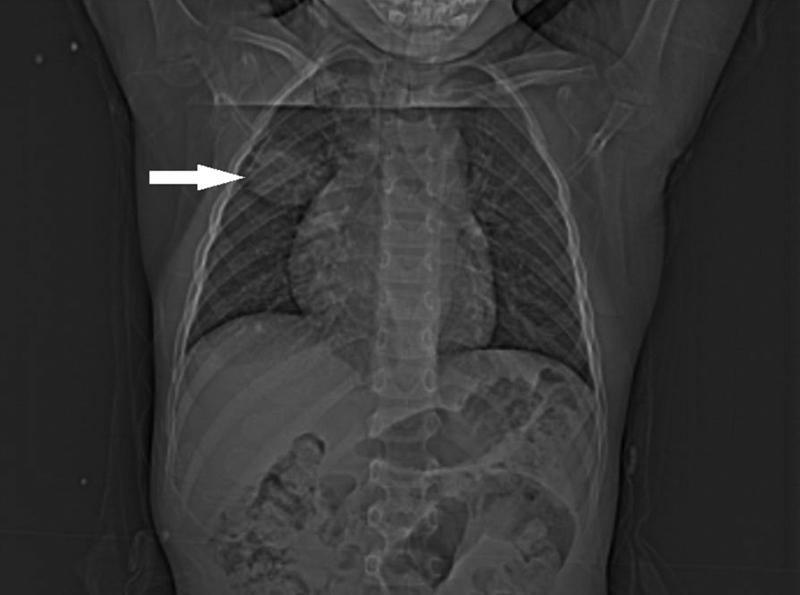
Chest X-ray image demonstrating a right middle pulmonary opacity (arrow).
